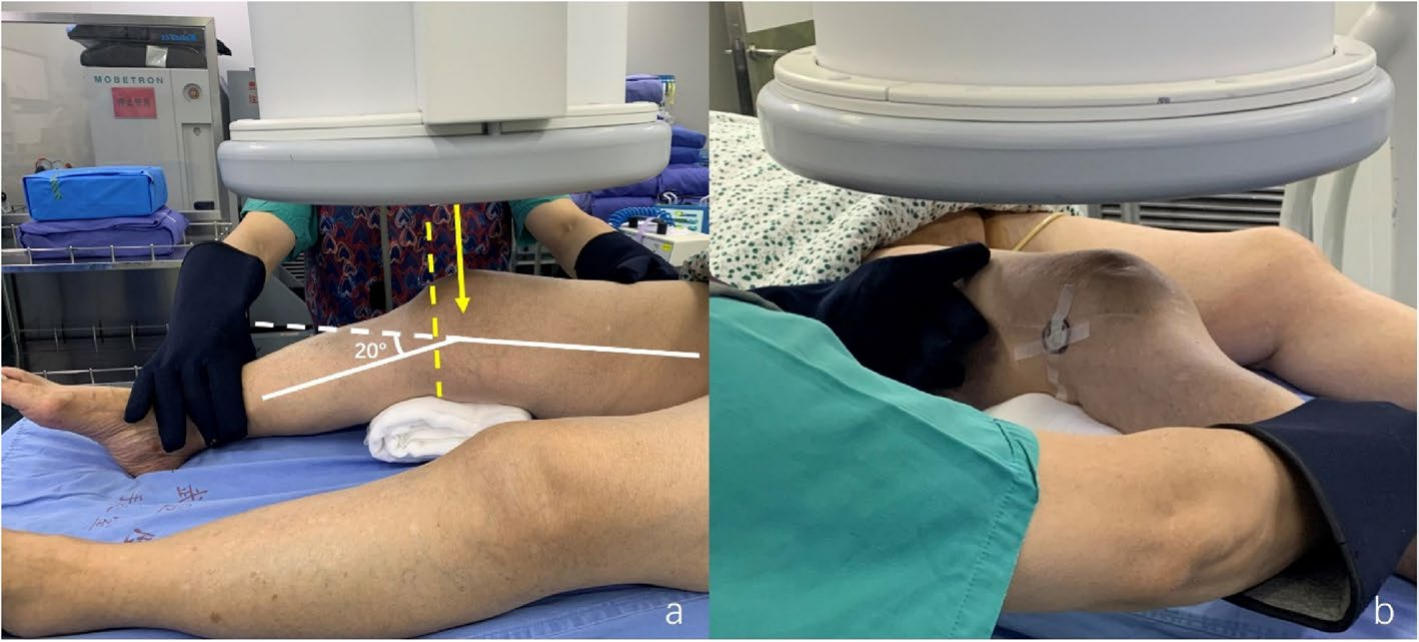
Conducting a standard valgus stress radiograph. a A cloth cushion was placed under the knee to form a 20° flexion. The surgeon kept the lower limb alignment neutrally and applied valgus stress manually. The X-ray beam was adjusted (yellow solid line) until it was parallel to the tibial plateau (yellow dotted line). b A 25 mm diameter round metal was placed in the center of the anteroposterior diameter of the distal femur to calibrate the magnification
